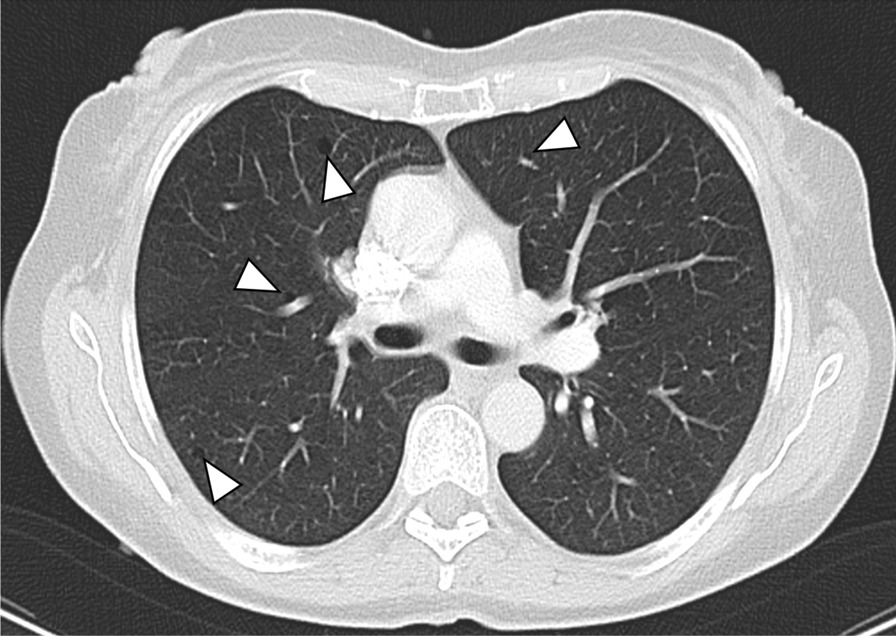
Transversal CT image of a 61-year old female non-smoker with several cysts in both upper lobes and the middle lobe (white arrowheads)
